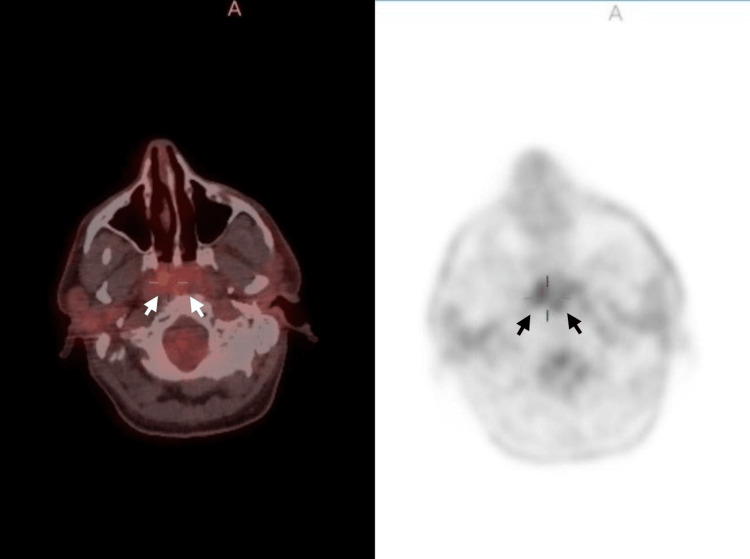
PET and fused PET/CT axial images demonstrating FDG uptake at the nasopharyngeal surgical bed, with higher uptake on the right side (SUVmax 4.2) and milder uptake on the left side (SUVmax 3.4)
